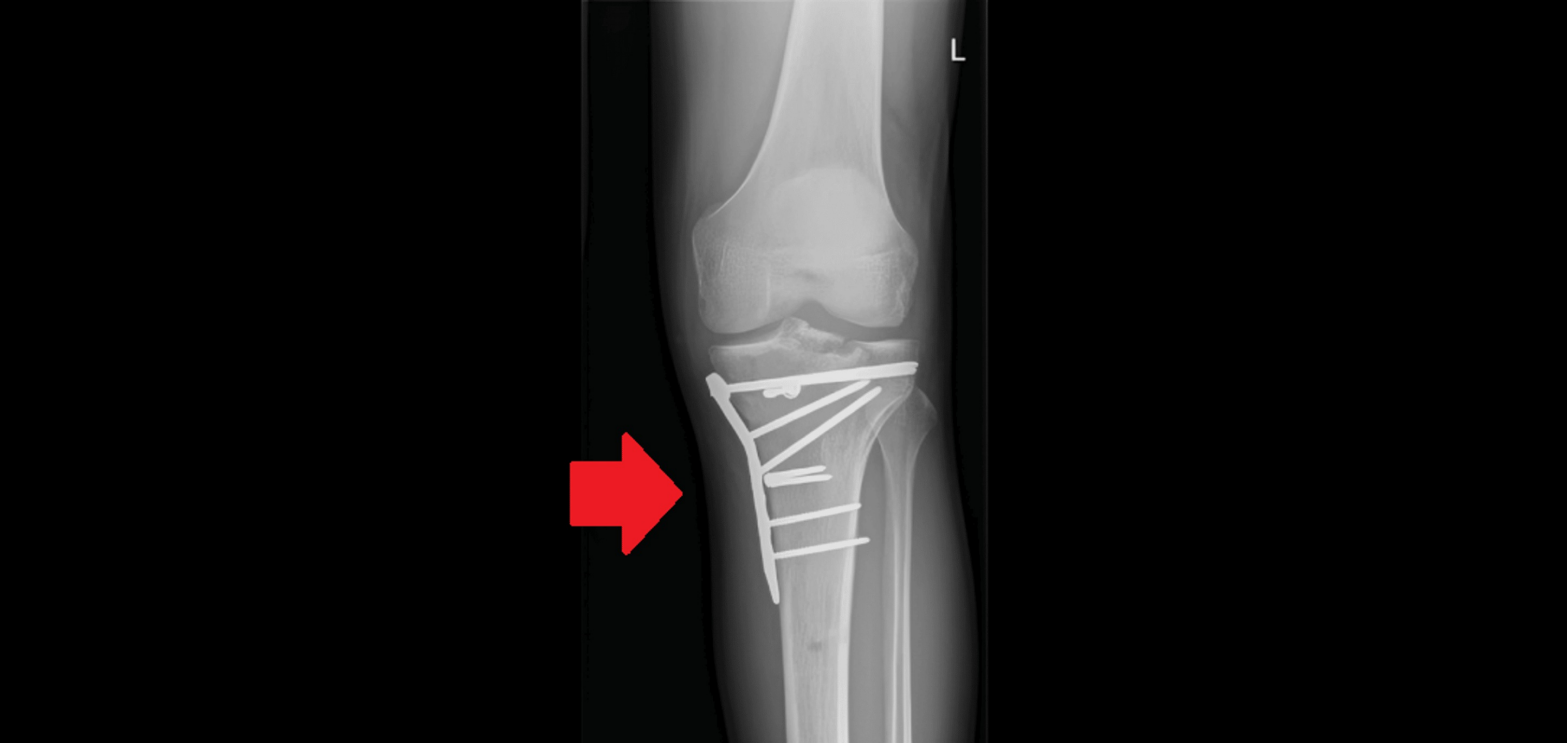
Radiography showing open reduction and internal fixation (ORIF) of the tibial plateau with the placement of an anteroposterior screw (anteroposterior view of the left knee)
